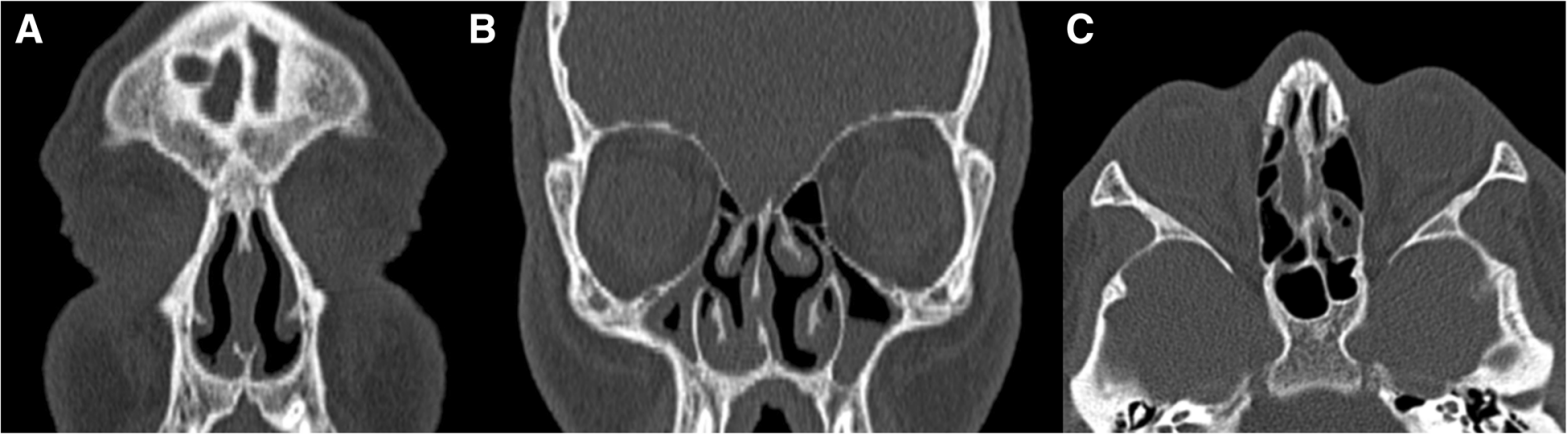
Coronal (a, b) and axial (c) reconstruction of paranasal sinus CT of a 21-year-old Down syndrome patient, depicting absent frontal sinuses and hypoplasia of the maxillary and sphenoid sinuses
